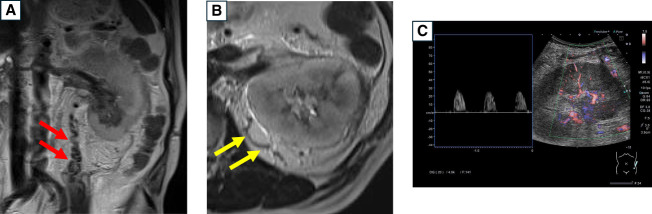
The findings of noncontrast MRI and Doppler ultrasound which suggest renal vein thrombosis. (A and B) magnetic resonance imaging showing enlargement of the (A) left testicular vein and (B) renal capsular vein. (C) Doppler ultrasound of the interlobar artery showing systolic phase-only blood flow.
